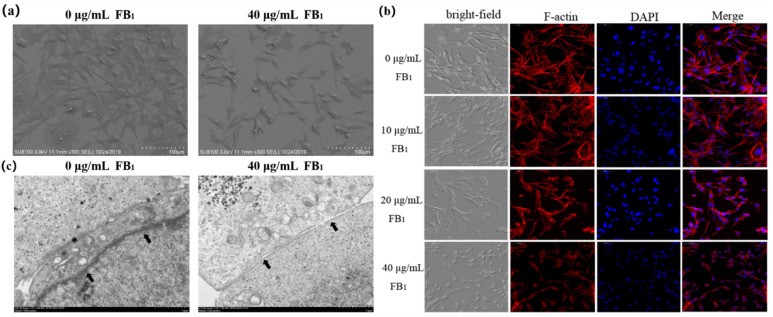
FB_1_ destroys the tight junctions of SUVECs. (a) Representative SEM images showing the ultrastructure of SUVECs (×300); (b) Representative phalloidin images showing microfilament skeleton of SUVECs (×200); (c) Representative TEM images showing the cell connection of SUVECs (×10,000); the destruction of tight junctions (black arrowheads) was observed. The data was expressed as mean ± SD.
